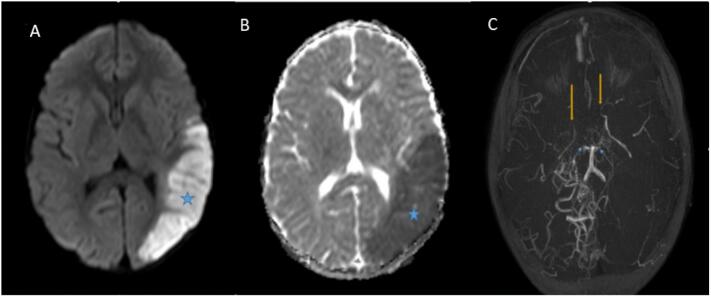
Selected axial brain images for our patient, Brain MRI and MRA (A) diffusion sequence demonstrates Left parieto-occipital cortical and subcortical area of high signal (blue star) with corresponding image low signal seen at (B) ADC sequence (Apparent diffusion coefficient) (blue star) in keeping with acute infarction. In MRA image (C) There is a significant narrowing of both middle cerebral arteries (MCA) M1 segments (yellow arrows) and a narrowing of P1 segments of both posterior cerebral arteries (PCA) (blue arrows).
